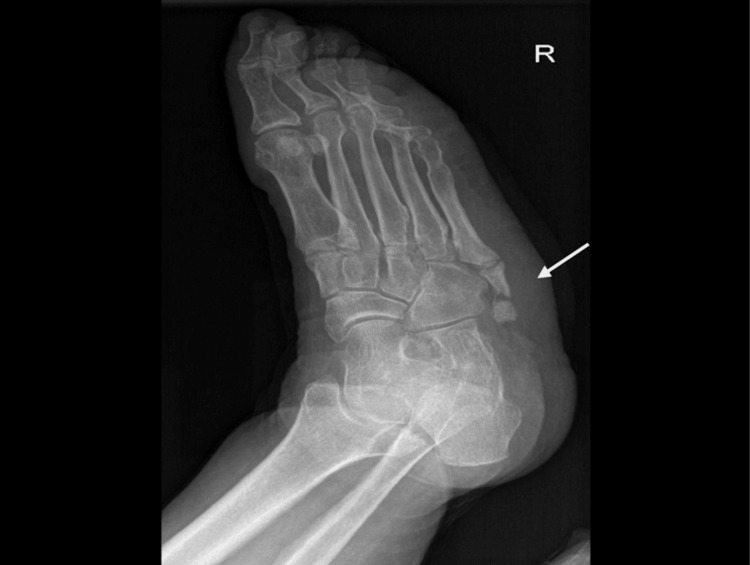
Right foot X-ray oblique view - accessory bone connected to the base of the fifth metatarsal bone.
